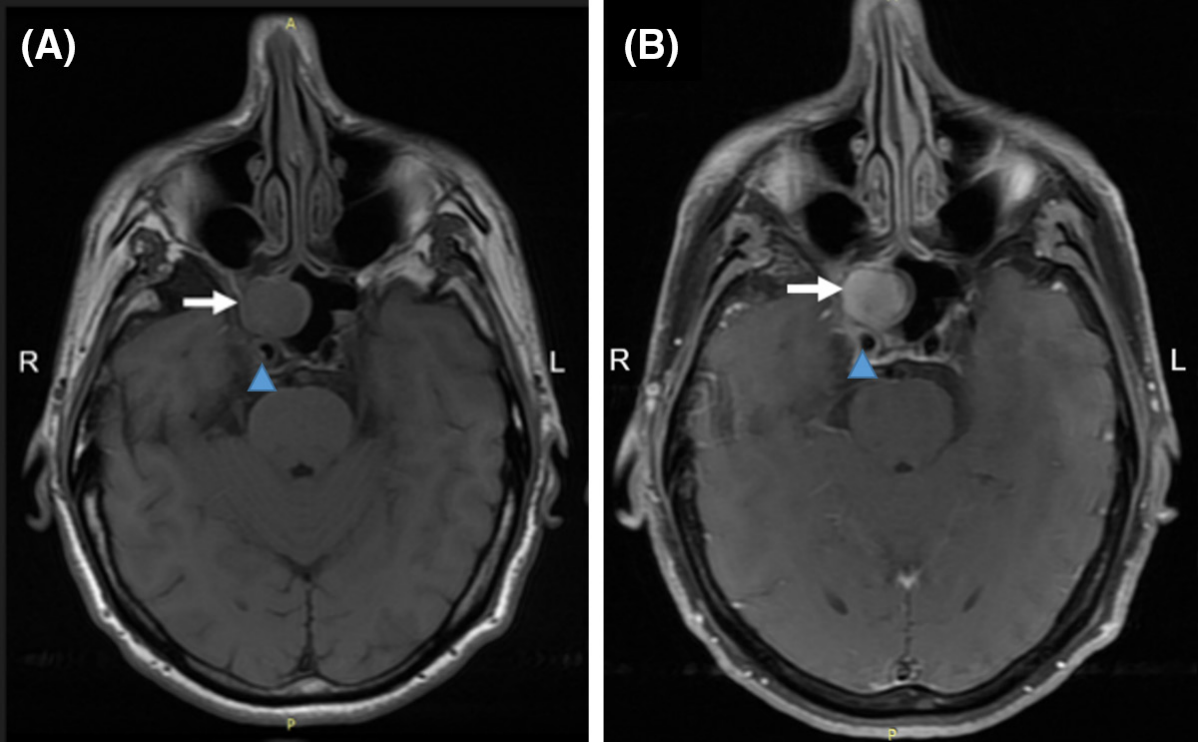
Sinus MRI. Tumor (white arrow) (2.0 × 1.8 × 1.6 cm) with invasion of surrounding structures. (A) Axial (T1W1 sequence), (B) Axial (T1W1 sequence with paramagnetic contrast agent administration). Blue arrowheads demonstrate the right ICA.
